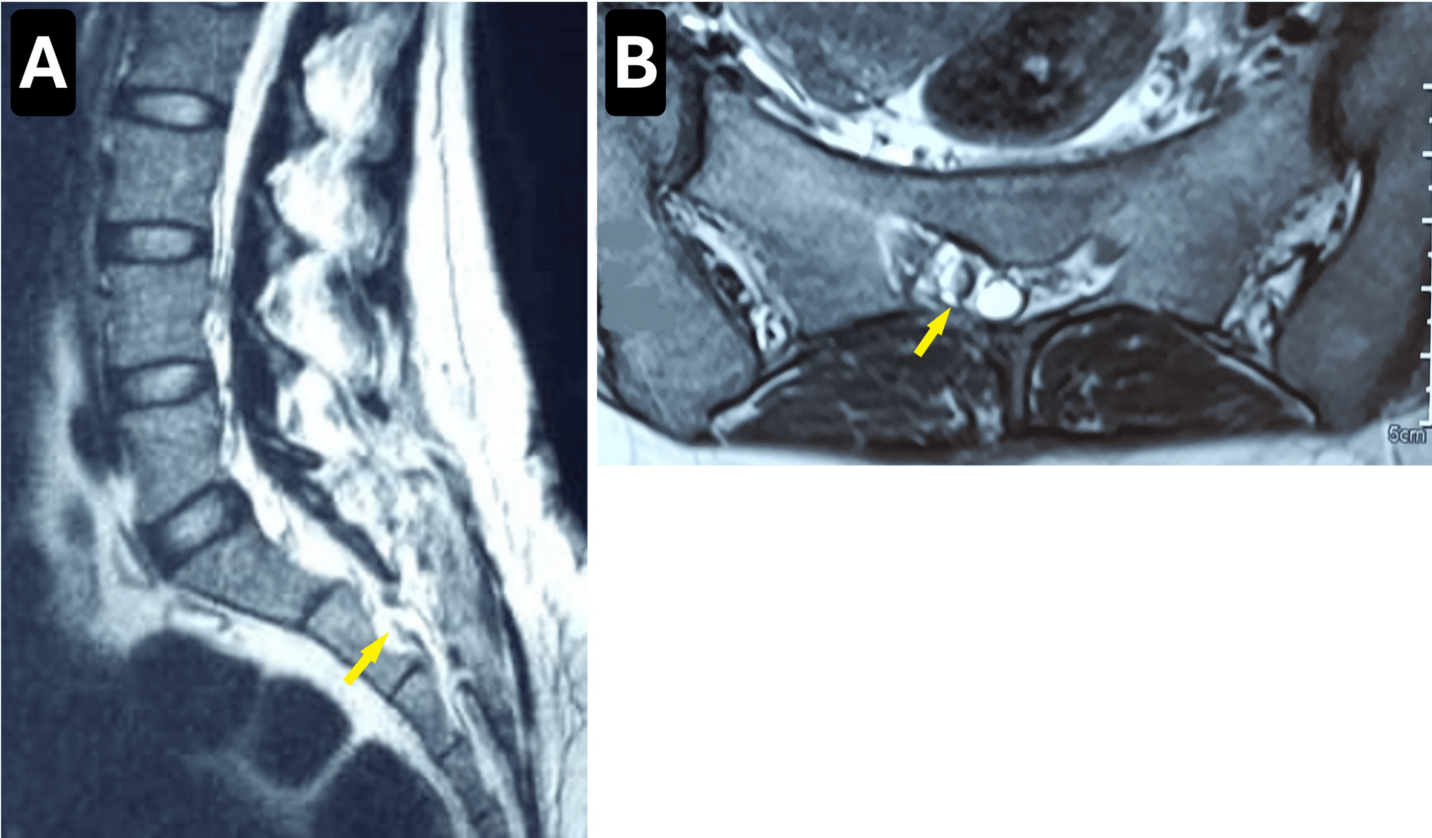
Postoperative MRI findings Sagittal (A) and axial (B) T2-weighted MRI scans obtained six months postoperatively show complete disappearance of the Tarlov cyst, with no evidence of recurrence or residual compression. Yellow arrows mark the previous location of the cyst.
